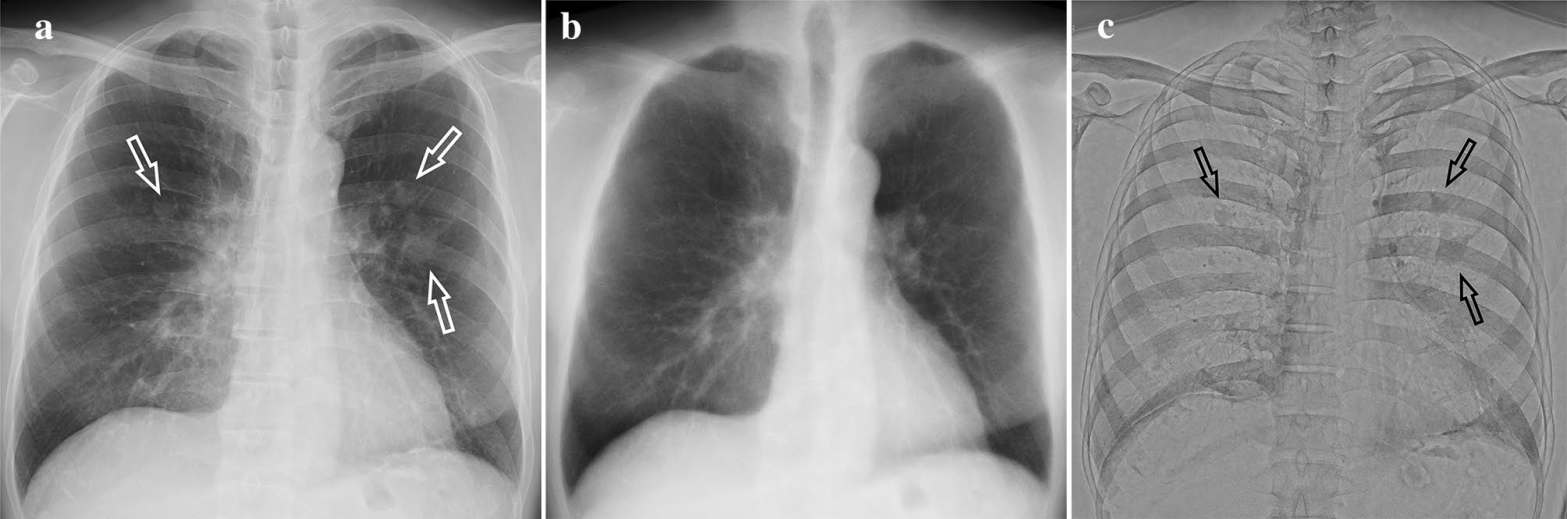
Male, 80 s, pleural calcifications. a Conventional radiography showed bilateral nodular shadows (arrows). b The soft tissue image showed no nodular shadows. c The bone image showed bilateral nodular shadows(arrows), which were determined to be pleural calcifications
